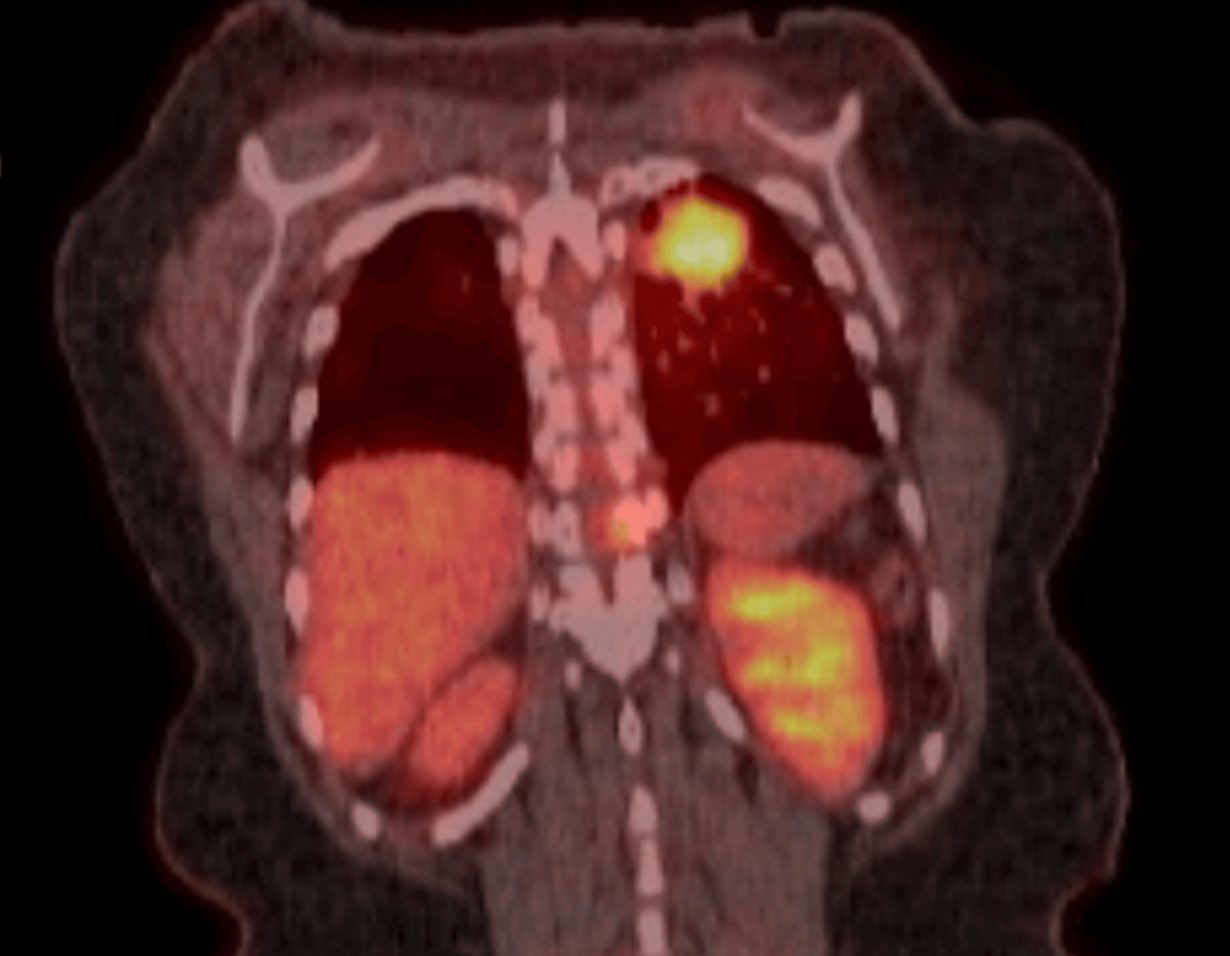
Positron emission tomography-computed tomography scan showing 2.9 x 2.4 x 2.8 cm FDG-avid right upper lung mass (SUV max 5.6)
